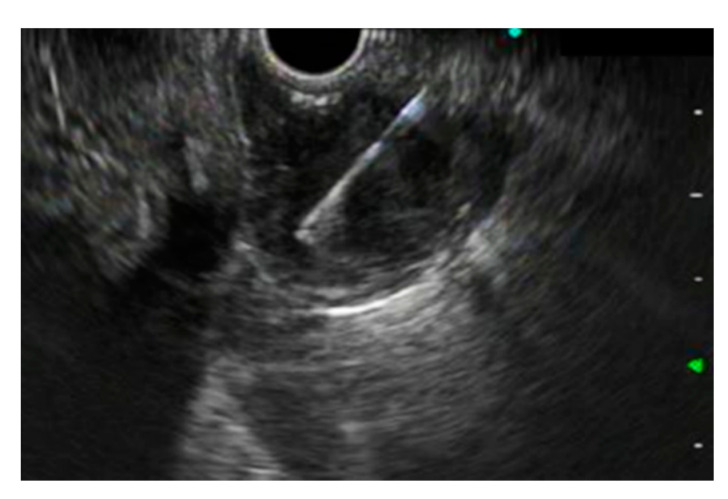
Endoscopic ultrasound-guided fine-needle aspiration (EUS-FNA) of a gastric subepithelial lesion.
